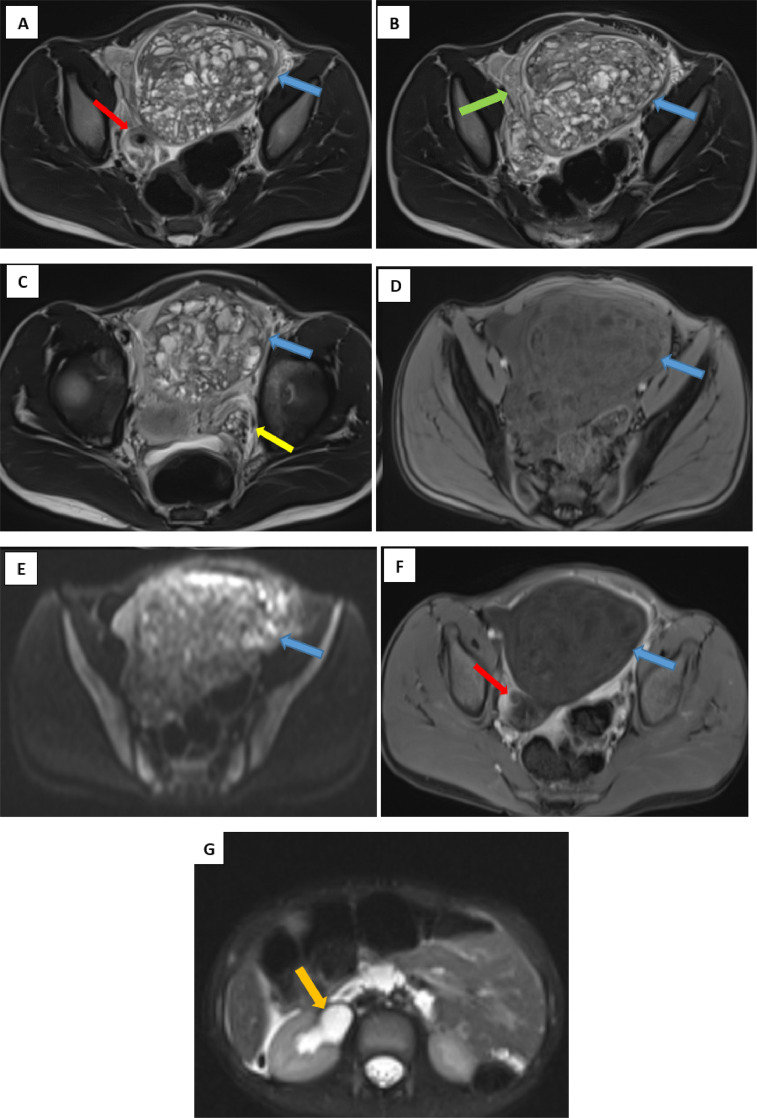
Abdominopelvic MRI. Axial views: T2-weighted sequence (A, B, C), T1-weighted sequence (D), diffusion-weighted imaging (E), contrast-enhanced T1-weighted sequence with fat suppression (F), and T2-weighted fat-saturated HASTE sequence (G). A large pelvic mass (blue arrow) is visualized, showing the "spurring" sign with the right ovary, which exhibits peripheral follicular distribution and stromal edema (green arrow). The mass is well-circumscribed, lobulated, and heterogeneous, with both cystic and solid components. It appears isointense on both T1- and T2-weighted images. Diffusion-weighted imaging reveals restricted diffusion, and there is an absence of enhancement of both the mass and the residual ovarian parenchyma following gadolinium injection. A twisted pedicle and ipsilateral fallopian tube are also noted (red arrow), along with upstream hydroureteronephrosis (orange arrow). The left ovary (yellow arrow) is of normal size and morphology, with multiple follicles.
